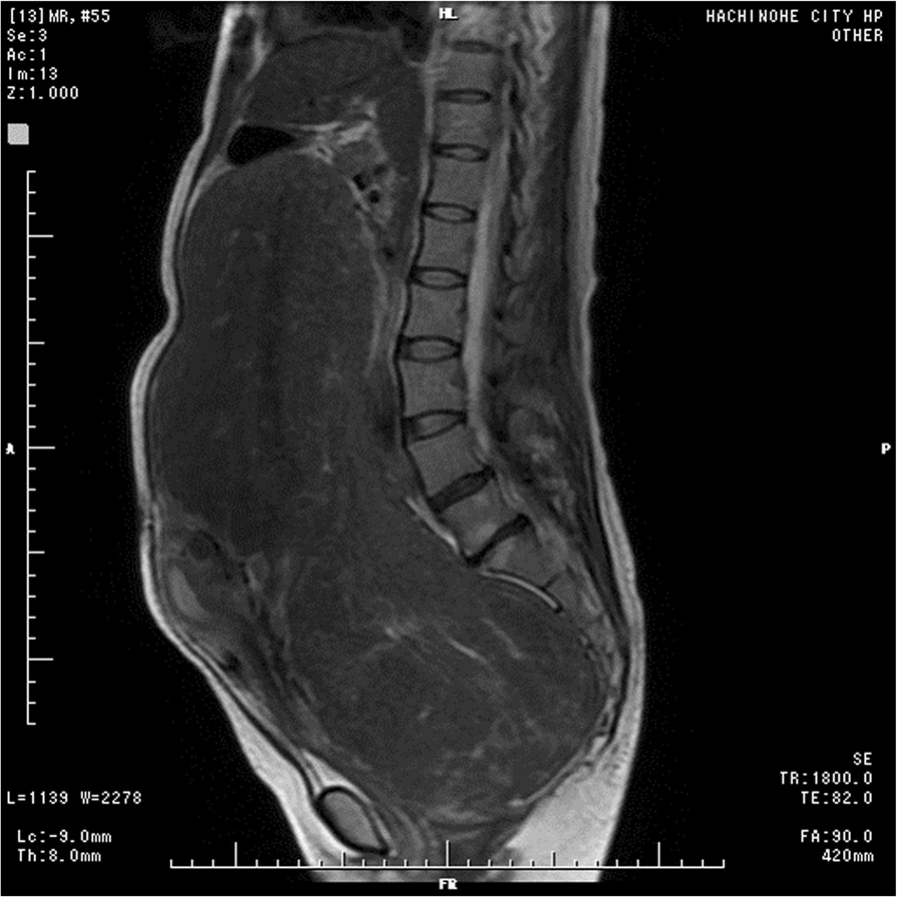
A sagittal T2-weighted magnetic resonance image of the uterus
